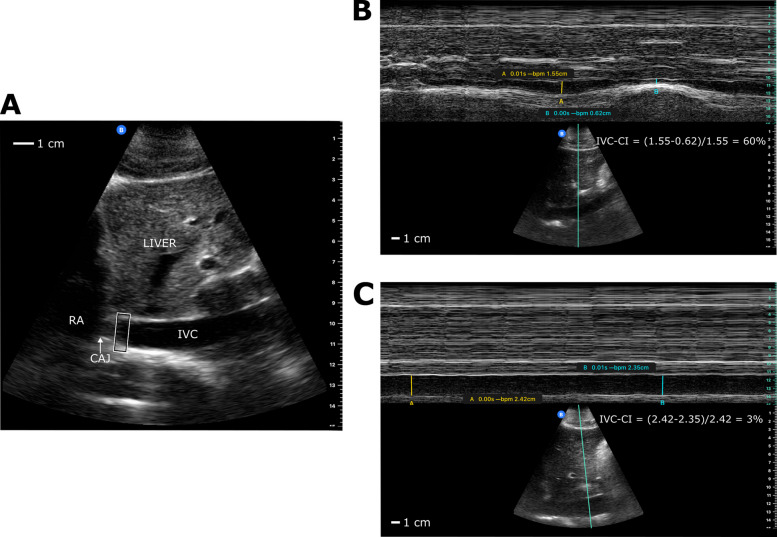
Point-of-care ultrasound (POCUS) images of the inferior vena cava (IVC). A A typical POCUS image of the IVC depicting the liver, right atrium (RA), IVC, and cavoatrial junction (CAJ). The box indicates the optimal position for IVC assessment, 1–2 cm from the CAJ. B IVC POCUS suggesting the absence of volume overload with an IVC diameter ≤ 2.1 cm and an IVC collapsibility index (IVC-CI) > 50%. (C) IVC POCUS suggesting the presence of volume overload with IVC diameter > 2.1 cm and IVC-CI < 50%
